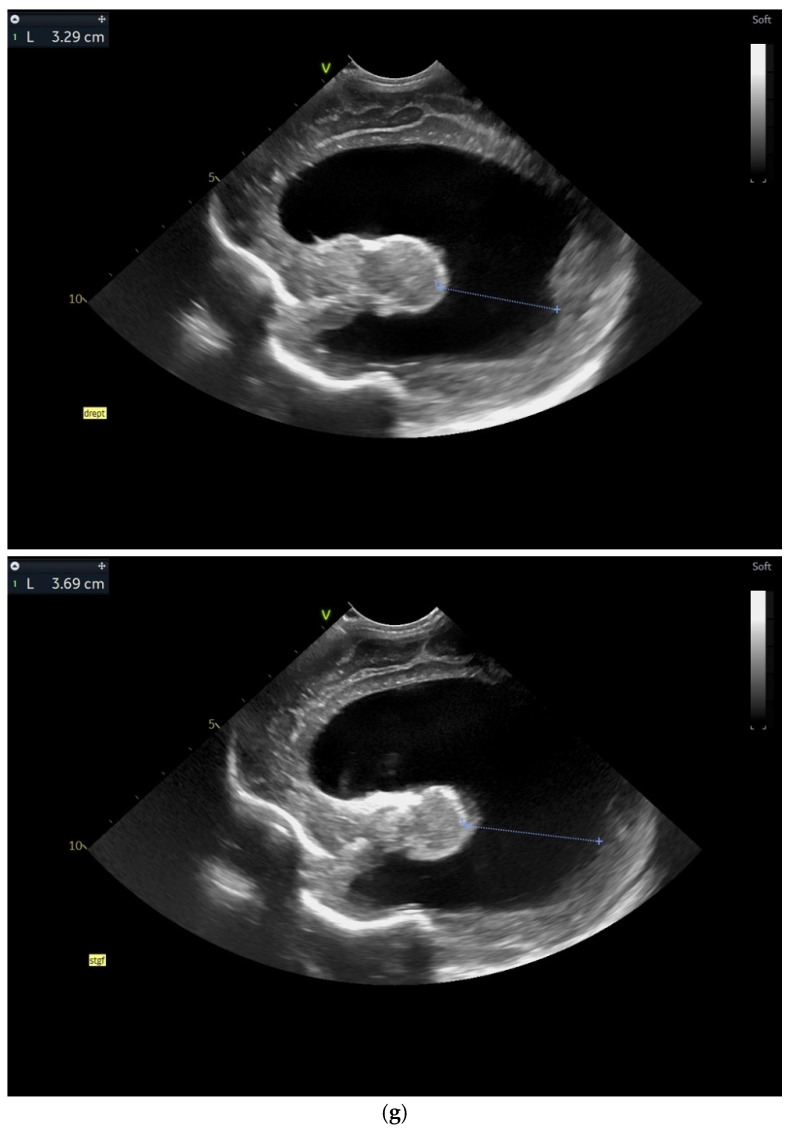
(a) Cranial ultrasound. Coronal view. Lateral ventricles and third ventricle enlargement. L1, L2—Levine index measurement. L3, L4—posterior horn of the lateral ventricles’ width measurement. L5—third ventricle width measurement (personal image collection). (b) Cranial ultrasound. Sagittal view. Enlarged third and fourth ventricles. L1, L2—third ventricle measurements. L3, L4—fourth ventricle measurements (personal image collection). (c) Cranial ultrasound. Sagittal view. Enlarged lateral ventricles. Up—left ventricle. Down—right ventricle (personal image collection). (d) Cranial ultrasound. Coronal view. Punctuate hyperechoic images were observed in the right lobe (personal image collection). (e). Up left—MRI T1 sequence. Up right—MRI T2 sequence—susceptibility weight imaging (SWI). Down—CT scan. Transversal view. Yellow arrows—multiple calcifications can be seen in the CT scan (personal image collection). (f) Cranial ultrasound. Coronal view. Lateral ventricles. L1, L2—Levine index measurement. L3, L4—anterior horn width measurement. Blue arrow—intraventricular shunt (personal image collection). (g) Cranial ultrasound. Sagittal view. Thalamo-occipital distance measurements. Up—right ventricle. Down—left ventricle (personal image collection).
